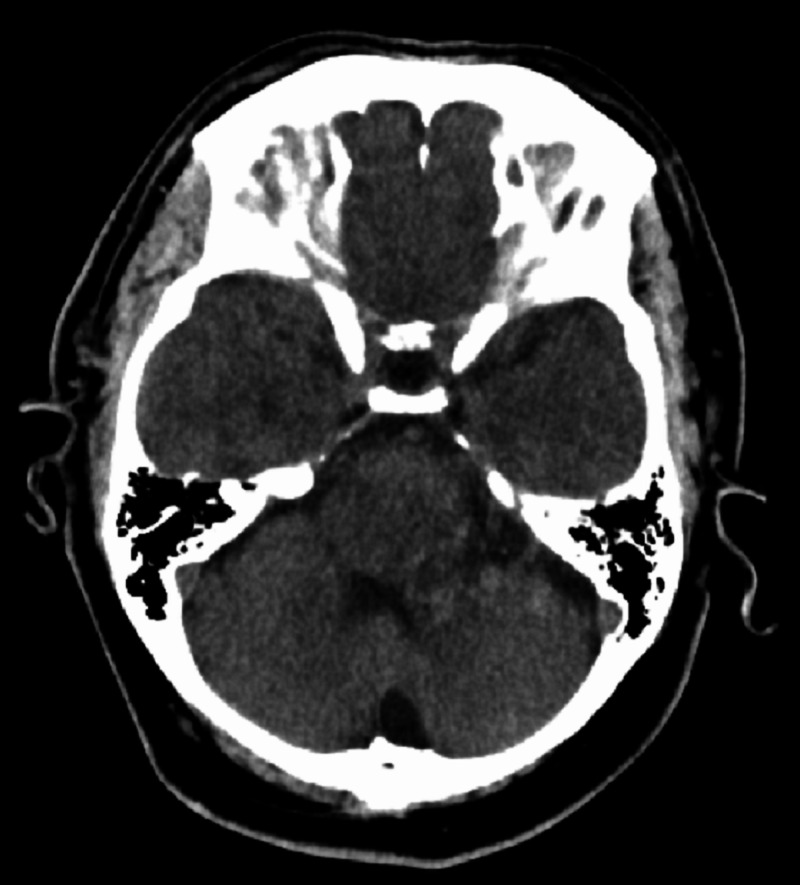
CT head without contrast, axial section
